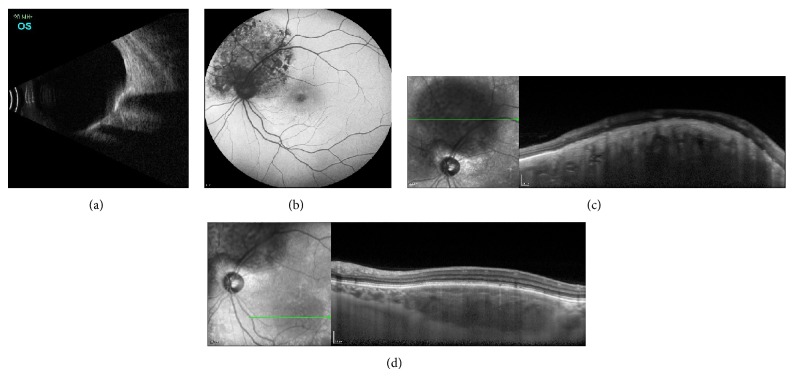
(a) Ultrasound showing a hyperreflective line with acoustic shadowing. (b) Autofluorescence of the left eye. The juxtapapillary tumor presents a hyperautofluorescence and hypoautofluorescence granular pattern. The paramacular tumor presents an isoautofluorescence pattern. (c) EDI-OCT of the juxtapapillary choroidal osteoma showing alterations on the external retina and the sponge-like structure. (d) EDI-OCT of the paramacular tumor shows an intact retina.
